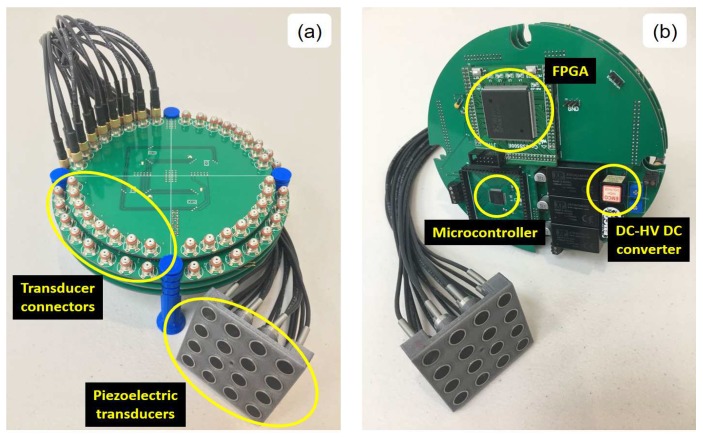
Ultrasound system designed for automatic pipeline inspection. (a) Top view and (b) bottom view.
